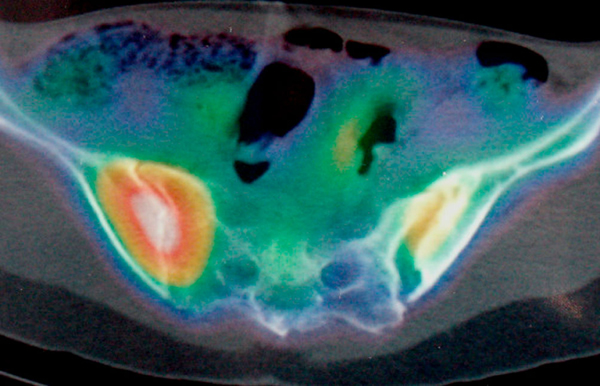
Single-photon emission computed tomography/computed tomography demonstrating a widened left sacroiliac joint with active bone remodelling and moderate inflammatory activity.
